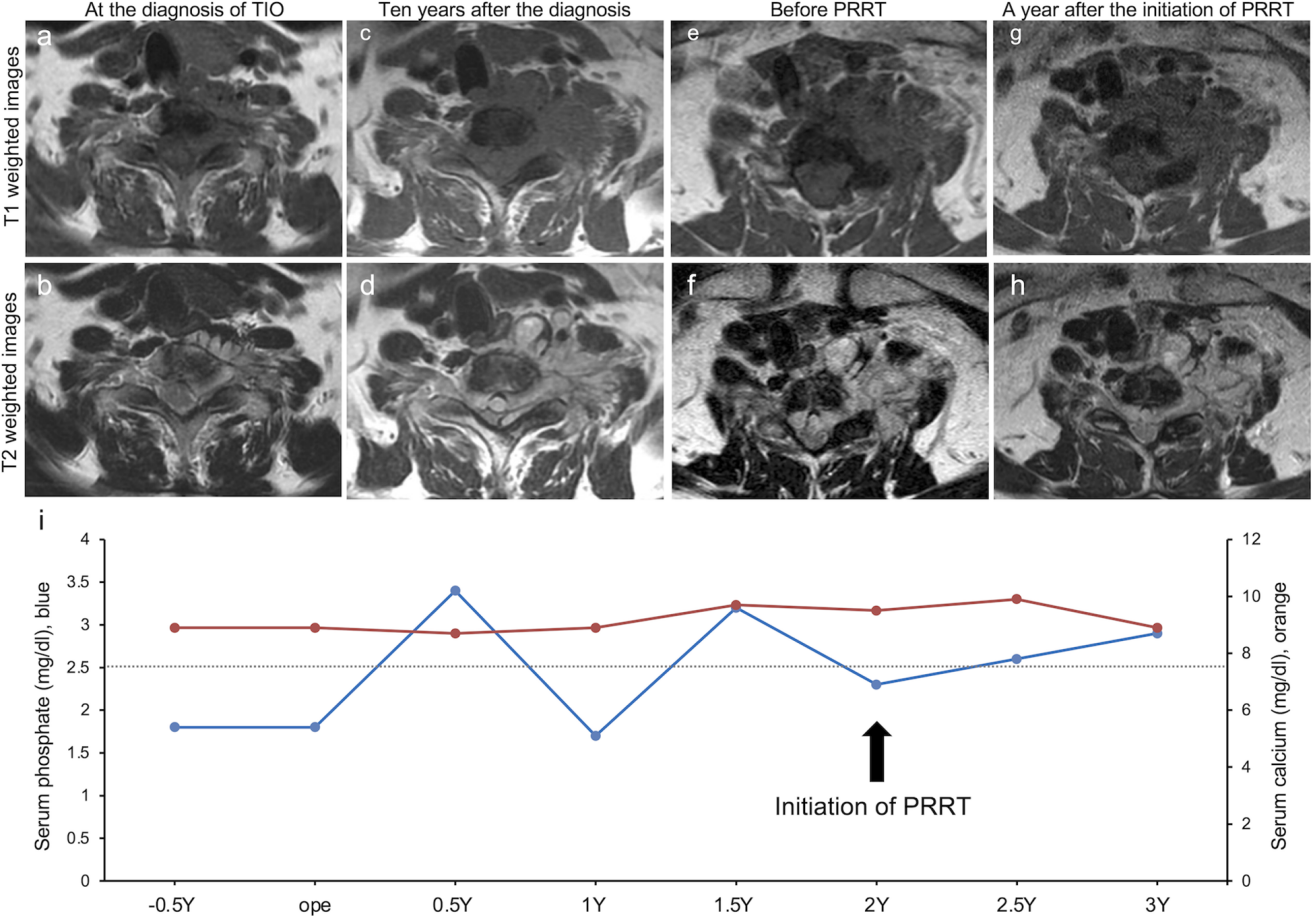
Longitudinal findings of medical images and laboratory tests. (a, b) Axial T1-weighted and T2-weighted imaging at the time of the diagnosis of tumor-induced osteomalacia (TIO) showing a 37-mm × 26-mm lesion at the right ventral aspect of the C7 vertebra. (c, d) Axial T1-weighted and T2-weighted imaging 10 years after the diagnosis showing a 56-mm × 39-mm lesion and marked tumor enlargement with extension into the spinal canal and compression of the spinal cord. (e, f) Cervical magnetic resonance imaging (MRI) (axial T1-weighted and T2-weighted images) immediately before peptide receptor radionuclide therapy (PRRT) initiation showing a 57-mm × 34-mm residual tumor. (g, h) Cervical MRI (axial T1-weighted and T2-weighted images) One year after PRRT initiation showing a 53-mm × 28-mm lesion. (i) Longitudinal laboratory tests of serum phosphate levels (blue, normal range: 2.5 mg/dL–4.5 mg/dL) and serum calcium levels (orange, normal range 8.5 mg/dL–10.2 mg/dL). Y, year(s).
